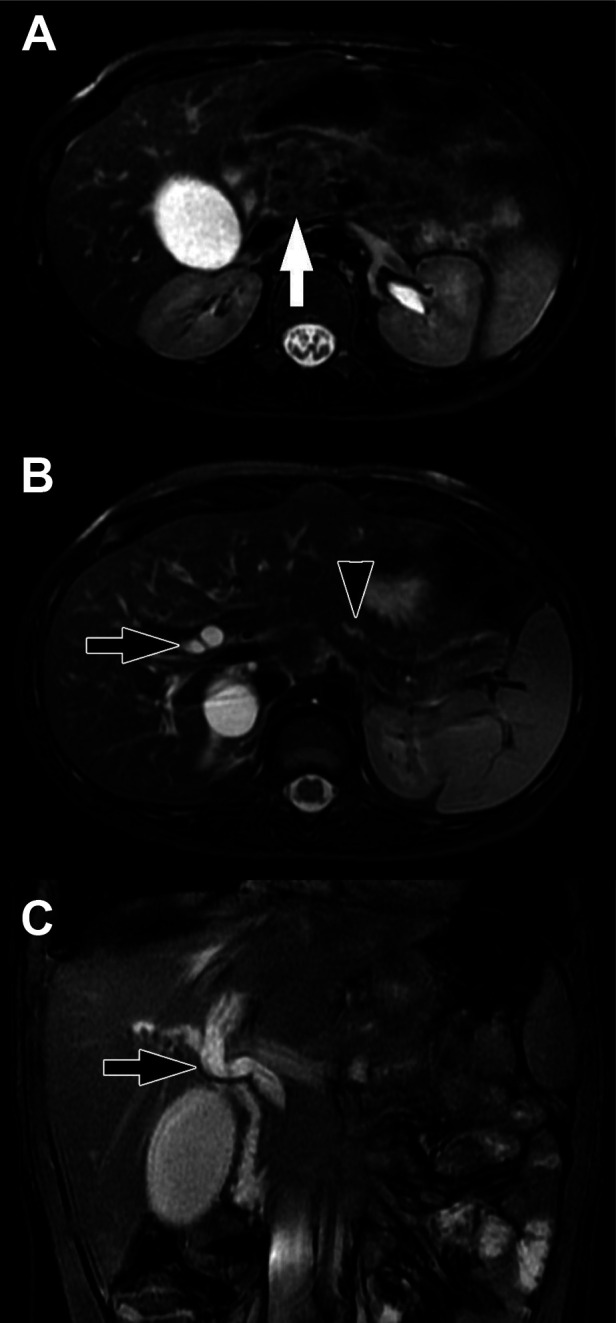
Focal pancreatitis. (A) Axial T2-weighted fat-saturated MFI shows heterogenous enlargement of the head of the pancreas (arrow). There is no substantial peripancreatic inflammation. (B) Axial T2-weighted fat-saturated MRI more cephalad in the same patient shows upstream bile duct dilation (black arrow) and dilation of the pancreatic duct (black arrowhead) due to obstruction by the process in the head of the pancreas. (C) Coronal balanced MRI in the same patient shows the extent of dilation of the bile ducts. The double duct sign of dilated pancreatic and bile ducts raises suspicion for a mass in the head of the pancreas.
